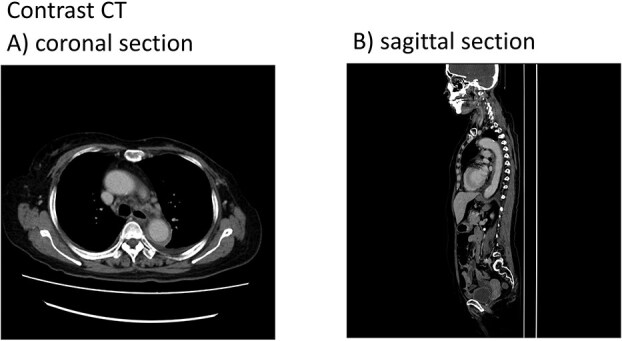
Contrast computed tomography findings: A) coronal section and B) sagittal section. Contrast computed tomography revealed wall thickening and increased density around the aortic arch and brachiocephalic artery.
